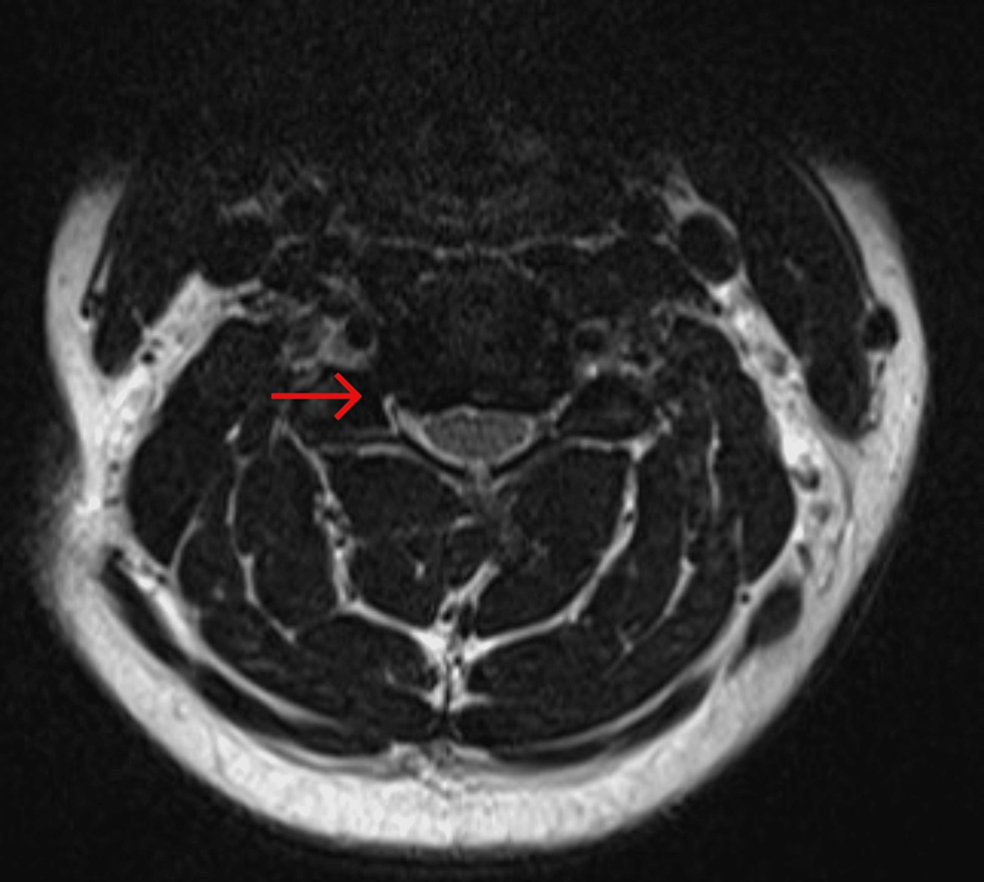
T2-weighted axial MRI of the cervical spine demonstrating severe right-sided foraminal stenosis at C4-C5 (red arrow)
